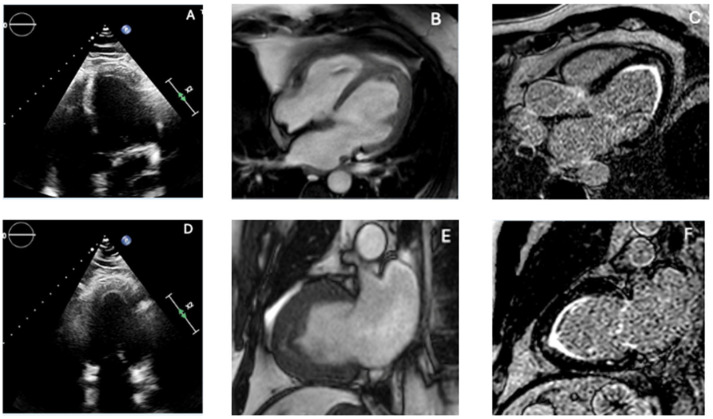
Multimodality imaging features of endomyocardial fibrosis. Combining echocardiography and CMR is essential to differentiate true apical obliteration from thrombus, hypertrophic variants, and infiltrative cardiomyopathies, as EMF shows a characteristic pattern of subendocardial fibrosis with or without overlying thrombus. (A,D) Transthoracic echocardiography reveals marked apical obliteration with increased endocardial echogenicity, reduced cavity size, and features consistent with restrictive physiology. (B,E) CMR cine images demonstrate apical thickening and impaired ventricular compliance, with reduction or loss of the apical cavity. (C,F) Late gadolinium enhancement (LGE) sequences show the typical subendocardial fibrotic pattern of EMF, frequently accompanied by an overlying apical thrombus, producing the characteristic “double V” sign. The combination of subendocardial LGE, apical cavity loss, and associated thrombus strongly supports EMF and differentiates it from ApHCM (compact hypertrophy with patchy LGE), LVNC (deep perfused recesses), and apical LVT (avascular mass without subendocardial fibrosis). Original images from our institution (single patient).
